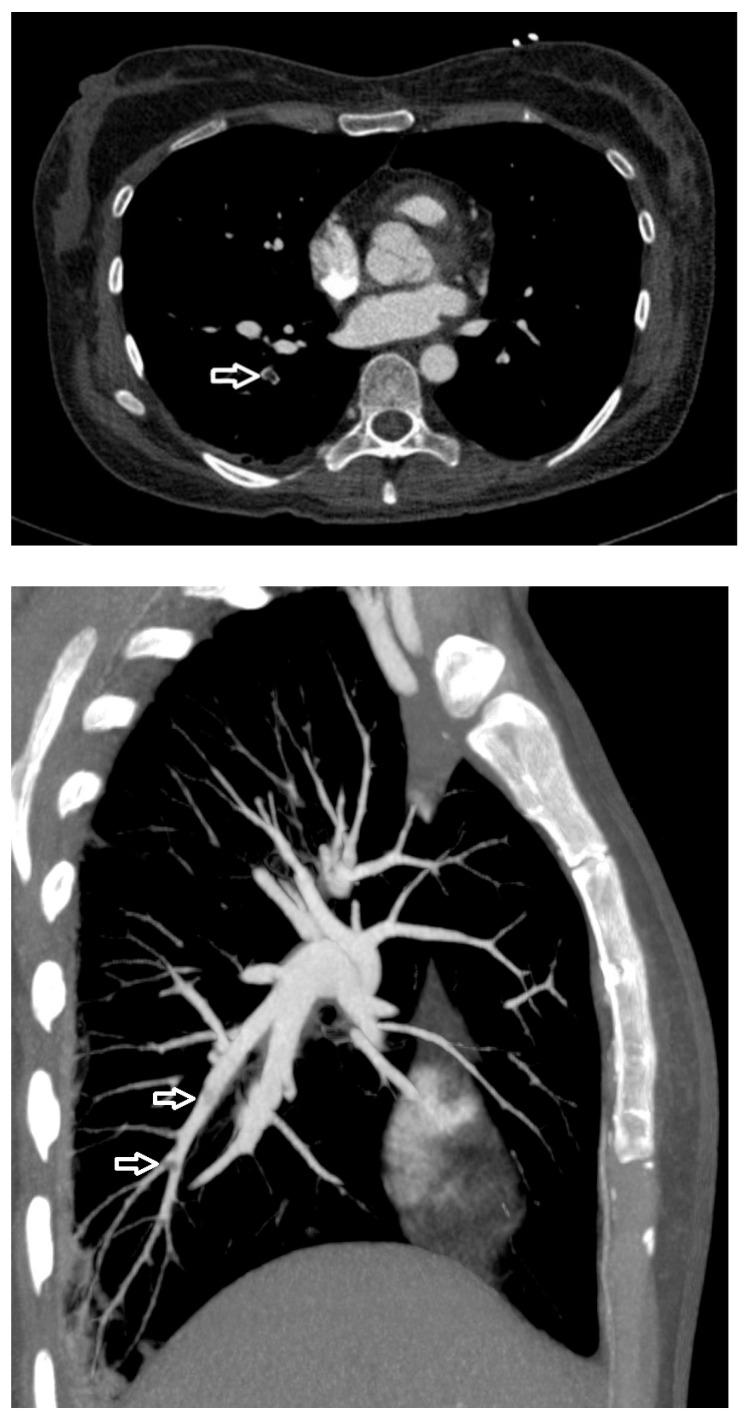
Computed tomography pulmonary angiography image shows filling defects (arrows) in the pulmonary artery, with a CT value of about −55 Hounsfield units (HU).
